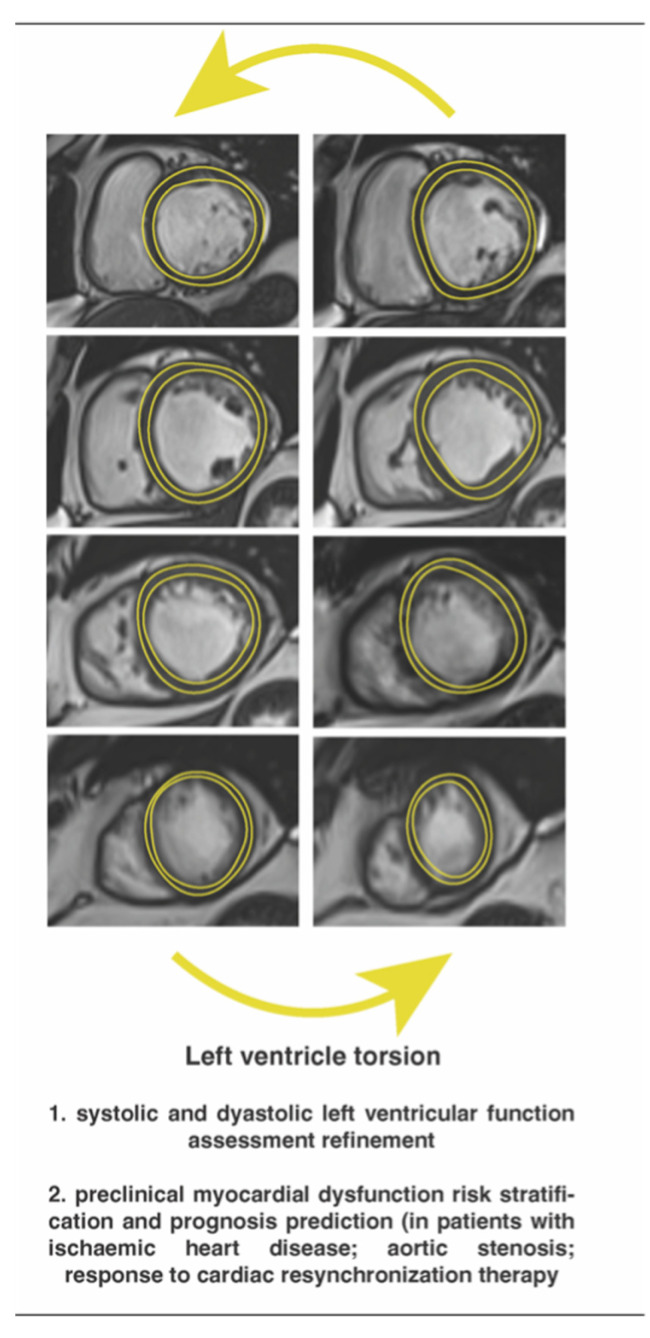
Left ventricle torsion by feature-tracking cardiac magnetic resonance imaging.
